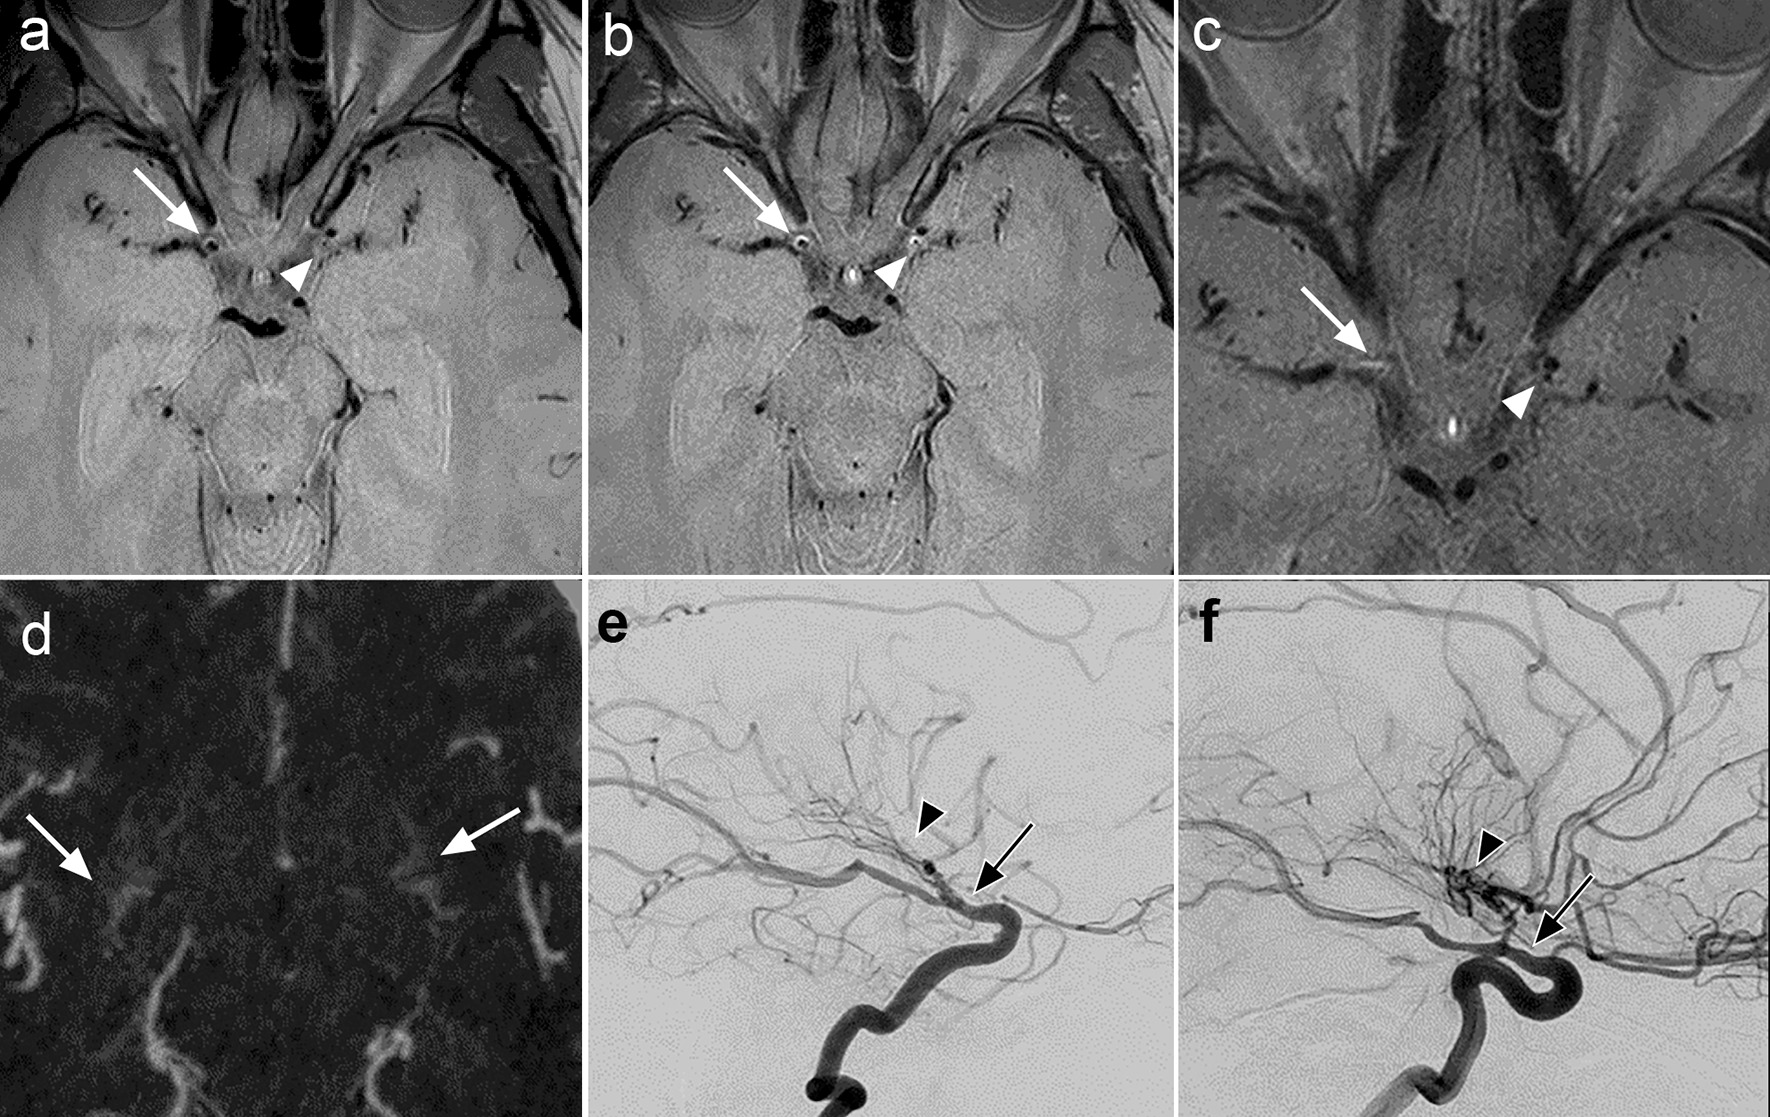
36-year-old female presented with right-sided weakness and border zone infarcts in the left cerebral hemisphere. The patient had a history of borderline hypertension and borderline hyperlipidemia. No other vascular risk factors. No oral contraceptives. No findings of inflammation on CSF testing. Panel to assess for hypercoagulability was negative. The best fit diagnosis was MMD. VWI without gadolinium demonstrates eccentric wall thickening of the distal right ICA (white arrow) and concentric thickening of the distal left ICA (white arrowhead) as well as negative remodeling of the involved segments (a). VWI with gadolinium demonstrates eccentric grade I enhancement of the distal right ICA corresponding to the region of thickening (white arrow) and concentric grade I enhancement (the predominant pattern was considered concentric throughout the involved area, though not uniformly concentric on the provided image) of the distal left ICA (white arrowhead) (b). The enhancement, in this case on both sides, is intermediate and approaches grade II, but was considered slightly less than the pituitary stalk and designated grade I. A one-year follow-up VWI exam shown for descriptive purposes shows bilateral progressive negative remodeling of the terminal ICAs, proximal M1 segments, and A1 segments without non-contiguous areas of vessel wall abnormality (not shown) as well as decreased vessel wall enhancement (c). An axial CTA MIP shows proliferation of bilateral lenticulostriate collateral arteries (white arrows) (d). One-year follow-up lateral DSA images on the right (e) and left (f) shows bilateral smooth tapered stenosis of the terminal ICAs with occlusion of the proximal middle and anterior cerebral arteries and proliferation of bilateral lenticulostriate collateral arteries, more marked on the left. No other steno-occlusive lesions were present in the cervical or intracranial arteries (not shown)
